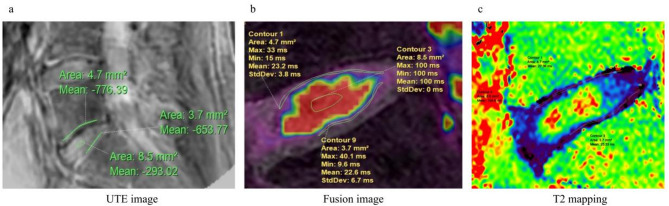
ROI sketching. From the L5-S1 vertebrae of a woman who underwent a lumbar MRI examination, 28 years old, no history of low back pain. a UTE Different echo subtraction image, the ROI initially outlined contains: contour 1, the area of the upper endplate, had an area of 4.7mm^2^; contour 3, the region of the nucleus pulposus, with an area of 8.5mm^2^; contour 9, the area of the lower endplate, has an area of 3.7mm^2^. The sketched ROI is then pasted into the fusion image and T2mapping image. b UTE subtraction sequence and 3D water sequence overlay image, The ROI copied from figure a contains: contour 1, an average UTE-T2 * value of 23.2ms; contour 3, with an average UTE-T2 * value of 100ms; contour 9, has an average UTE-T2 * value of 22.6ms. c T2 mapping sequence image, The ROI location and area outlined are consistent with those in a; contour 1, a mean T2 value of 27.36ms; contour 4, a mean T2 value of 104.6ms; contour 3, an average T2 value of 25.33ms
